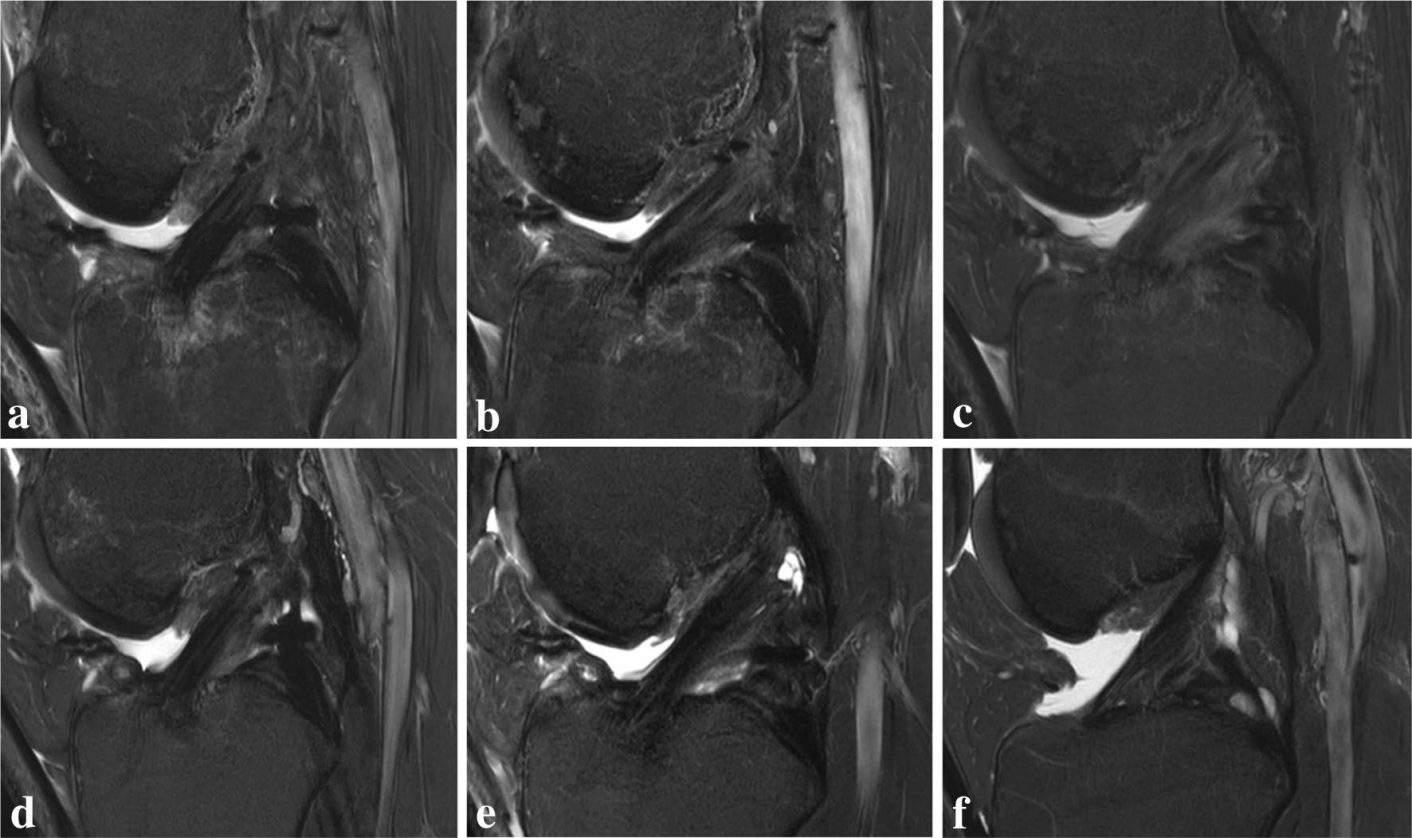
The maturation process of the anterior cruciate ligament autograft exhibits a significant MRI signal change from hypo-intense at (A) 6 weeks postoperatively to more hyper-intense at (B) 3- and (C) 6-months after ACL-R indicating greater disorganization of collagen tissue and higher water content. The MRI signal intensity decreases thereafter at (D) 1- and (E) 2-years post ACL reconstruction to approximate the signal of a native ACL (F). The distal native ACL revealed a more hyper-intense signal compared to the proximal and mid-substance ACL
